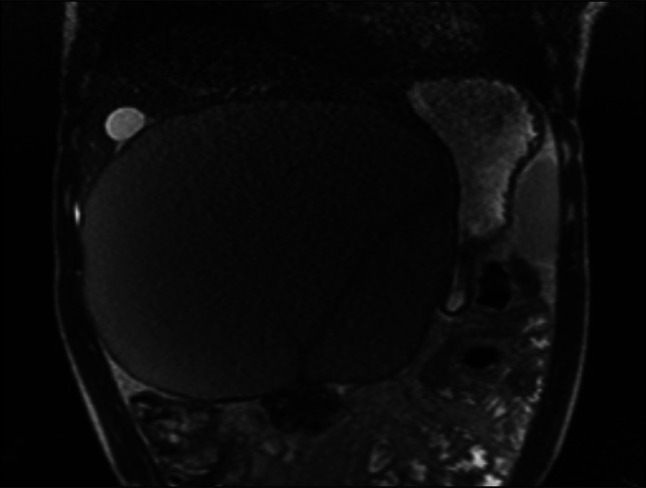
Magnetic resonance imaging of the abdomen with intravenous contrast in coronal and axial view showing a large subhepatic cystic mass, measuring 23.0 × 16.0 × 21.0 cm, which abuts the liver along its superior border.
